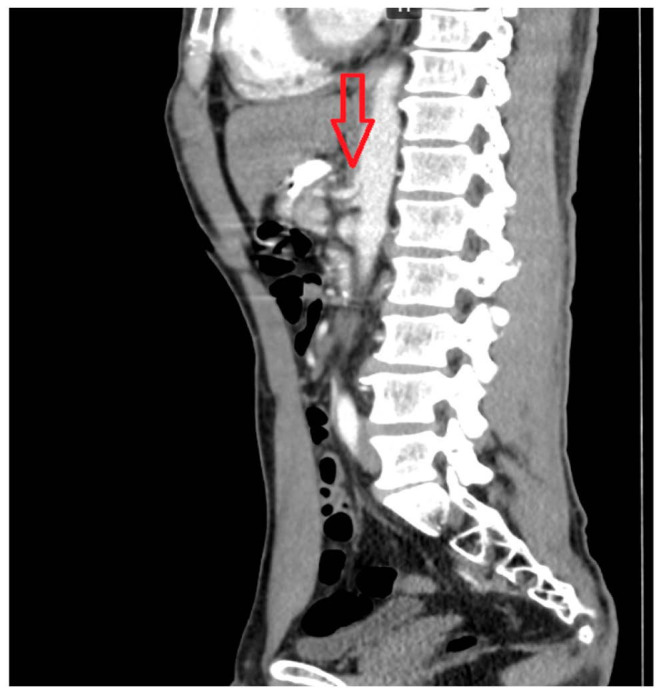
Contrast-enhanced multislice computed tomography (MSCT) of the abdominal organs demonstrating extravascular compression of the celiac trunk by the median arcuate ligament of the diaphragm (arrow).
